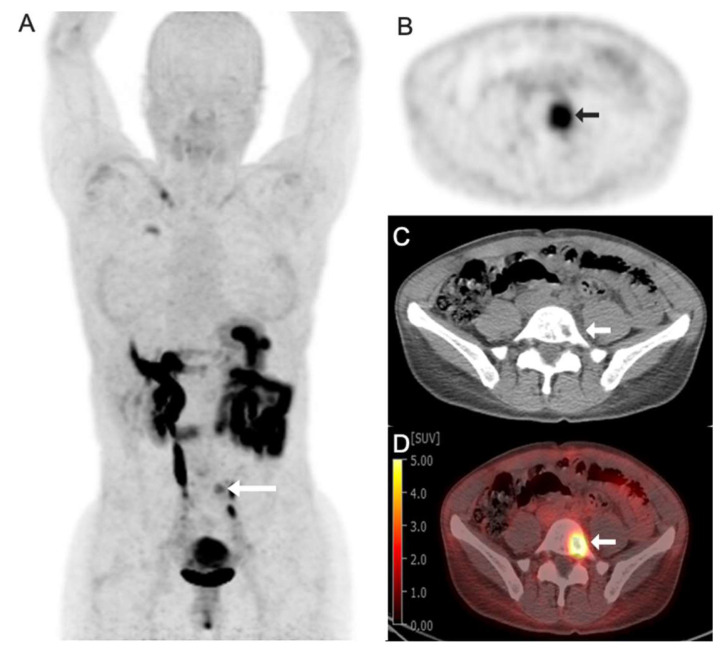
Images obtained from a 42-year-old woman with gastric cancer with focal radiotracer uptake in left L5 vertebral body lytic metastasis (arrows). (A) Fluorine 18 (^18^F)-labeled fibroblast activation protein inhibitor-74 (FAPI-74) maximum intensity projection image (MIP); (B) axial PET image; (C) axial CT image; (D) axial fused ^18^F-FAPI-74 PET/CT image with a Standard Uptake Value (SUV) max of 7.0.
